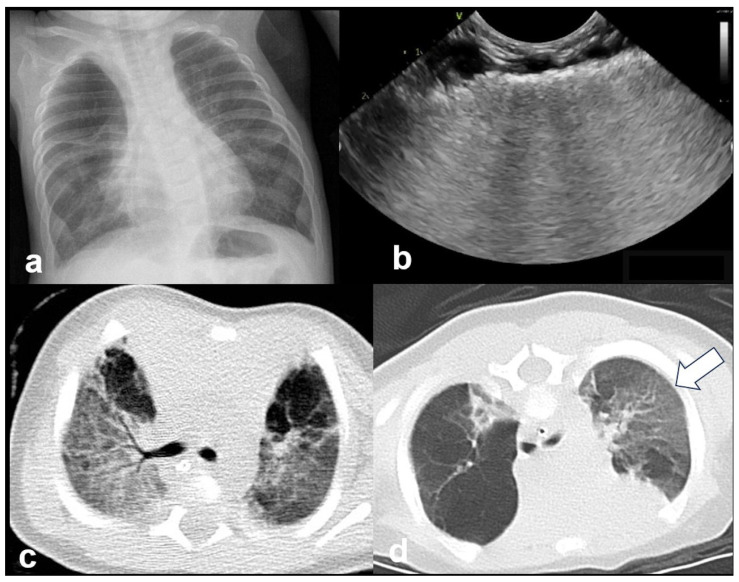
Bronchopulmonary dysplasia: (a) CXR; (b) LUS: abnormal thickening of pleural line; (c,d) CT. The main findings from all methods include lung parenchymal changes unequally distributed and contiguous to spared areas (arrow in (d)).
